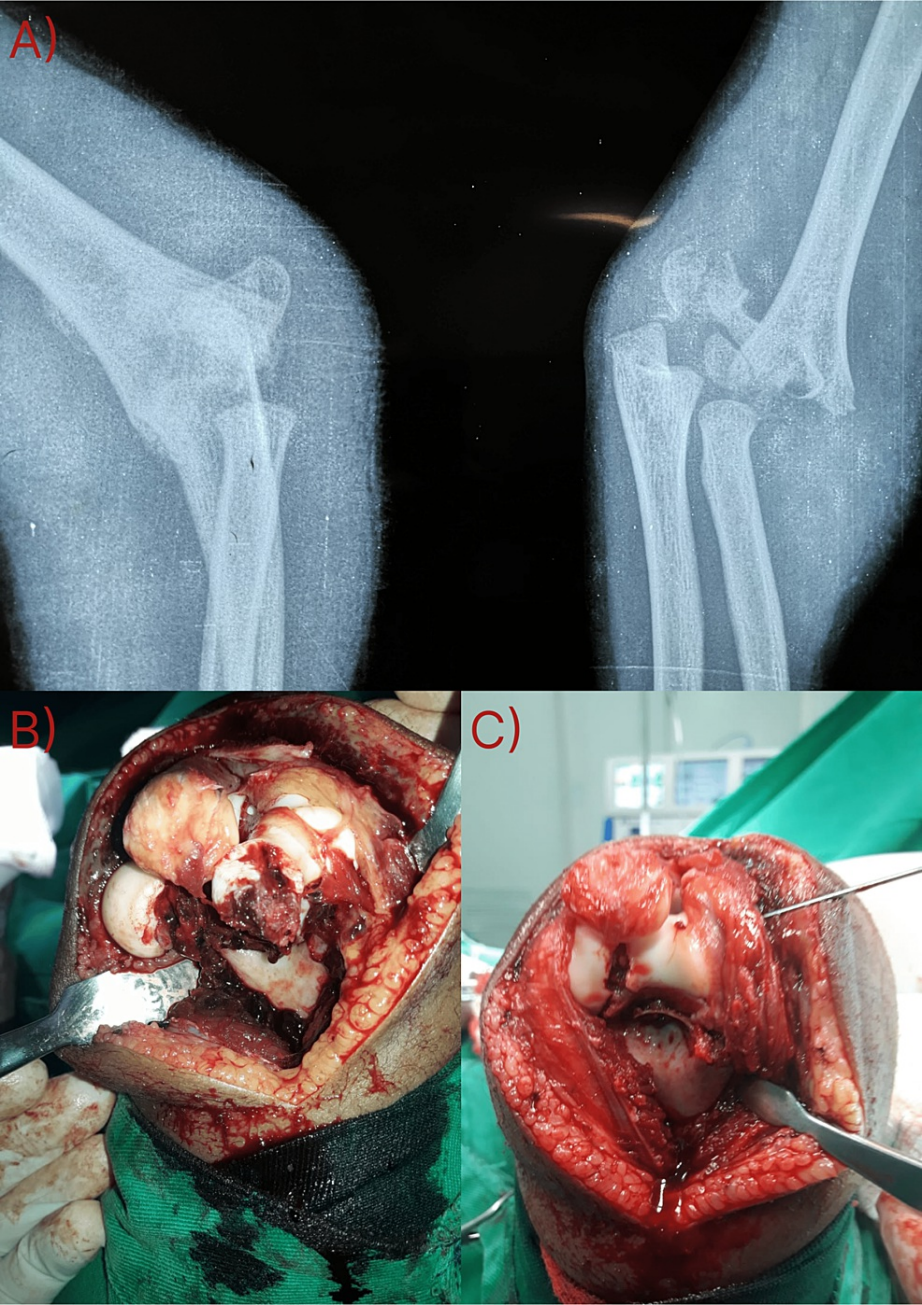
(A) Initial radiograph of the elbow showing a supracondylar humeral fracture with joint involvement, and (B and C) intraoperative image of a complex injury treated with anatomical reduction with lateral pinning
